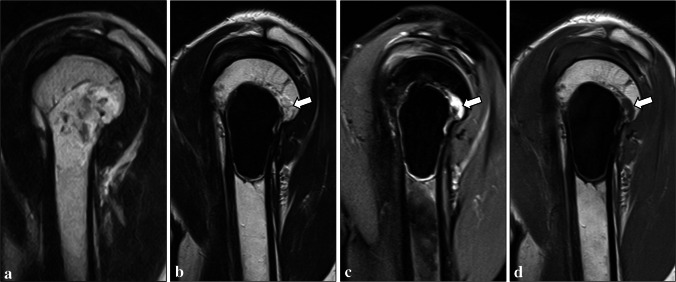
Residual tumor in a 57-year-old woman. On preoperative sagittal T2-weighted MRI sequence, an ACT of the proximal humerus could be seen (a). After curettage and cementation, a residual cartilaginous lesion (arrows) was identified on sagittal T2-weighted MRI sequences without (b) and with (c) fat suppression and on sagittal T1-weighted sequence (d), respectively. It was monitored through repeated MRI scans and showed no increase in size during a follow-up of 5 years
